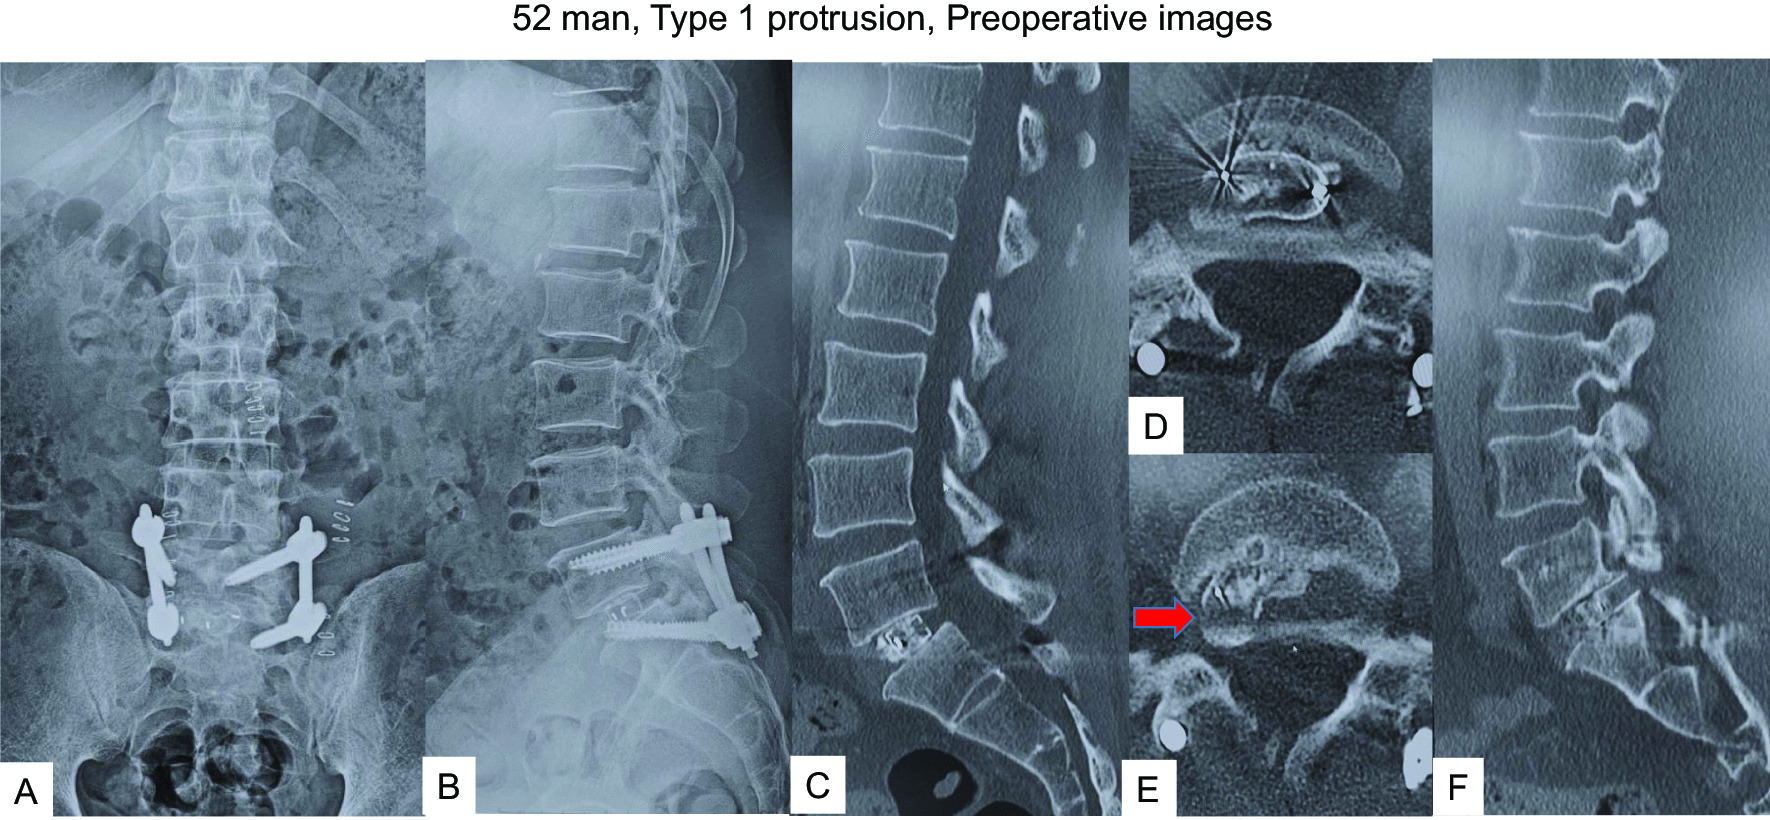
52 man, Type 1 protrusion, images before revision surgery A Postoperative anteroposterior radiogram, B Postoperative lateral radiogram, C Sagittal reconstruction CT at one month follow-up, D Axial CT at L5/S1 at one month follow-up, E Axial CT at L5/S1 at one year follow-up, F Sagittal reconstruction CT at one year follow-up. Red arrow shows a protruded cage
